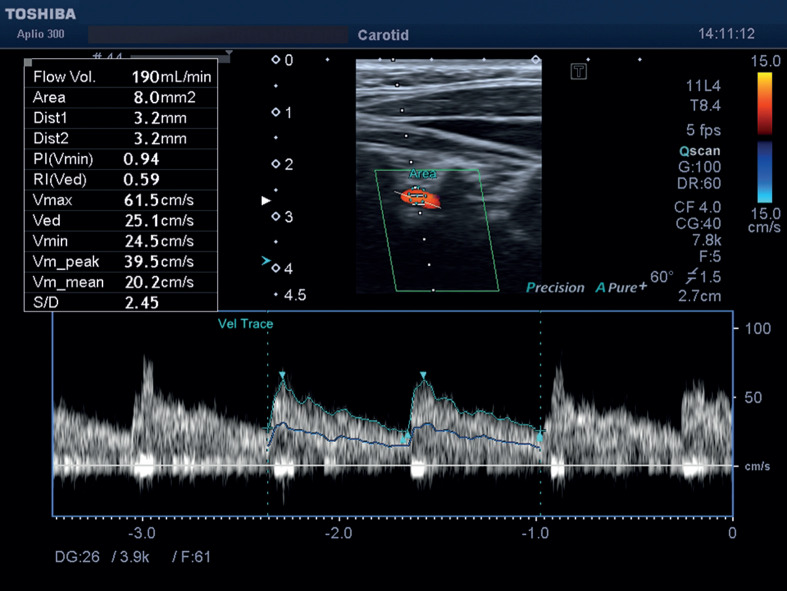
The left vertebral artery with normal flow parameters, flow volume, and diameter is observed with Doppler ultrasonography exam.
